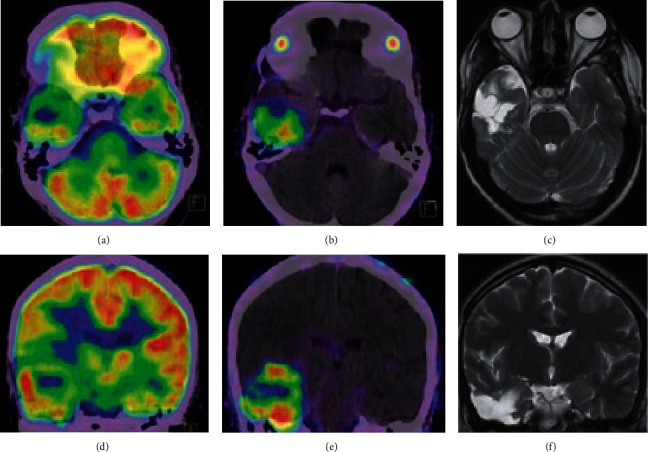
^18^F-FDG and ^68^Ga-PSMA PET imaging of representative GBM. (a) Axial ^18^F-FDG PET/CT. (b) Axial ^68^Ga-PSMA-11 PET/CT. (c) Axial T2-weighted MRI. (d) Coronal ^18^F-FDG PET/CT. (e) Coronal ^68^Ga-PSMA-11 PET/CT. (f) Coronal T2-weighted MRI. Reproduced with permission from Figure 1 in Sasikumar et al. [42].
